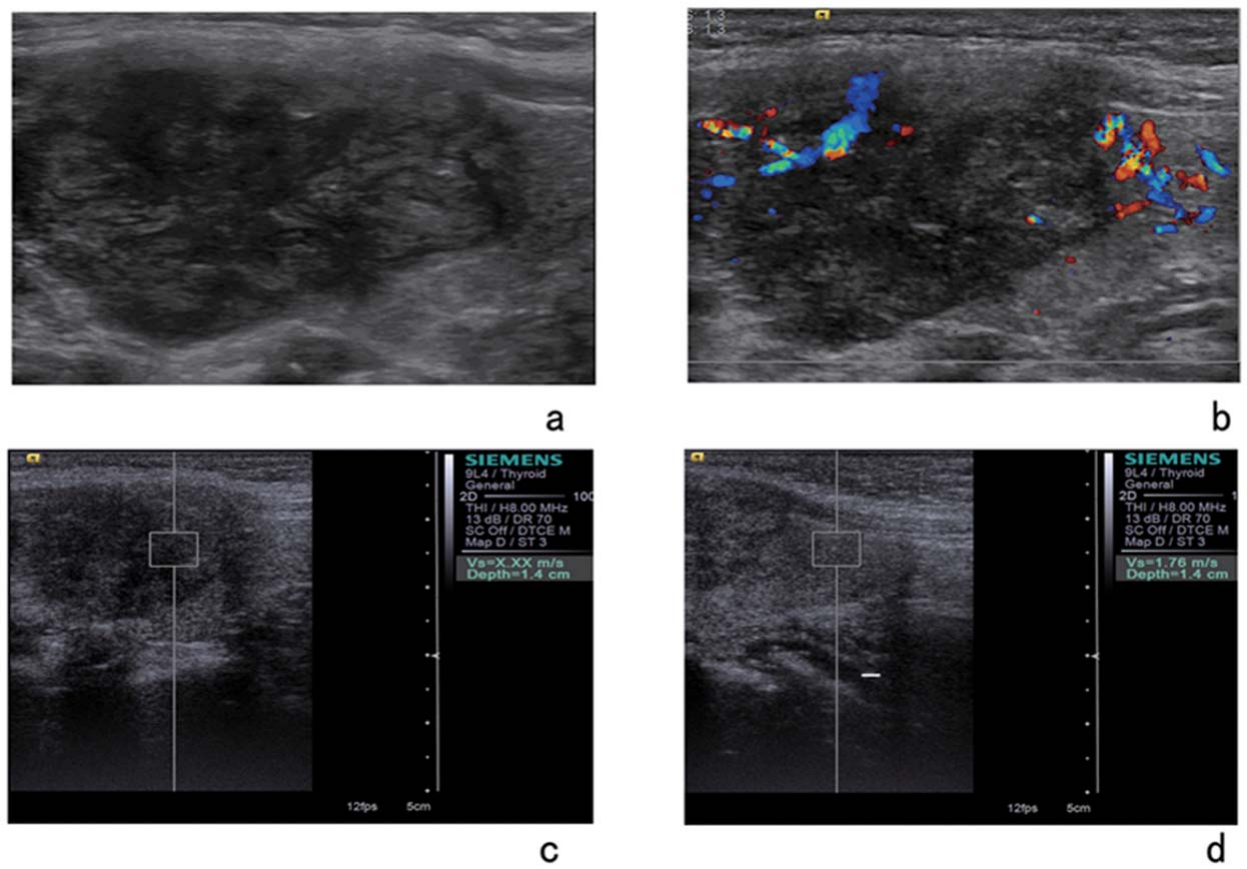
A 59-year-old woman with a solid lesion of papillary carcinoma. Gray-scale image (a) and color Doppler image (b) of the nodule. VTQ of the nodule shows the SWV is displayed as x.xxm/s and the SWV of the adjacent thyroid tissue (d) is 1.76 m/s.
